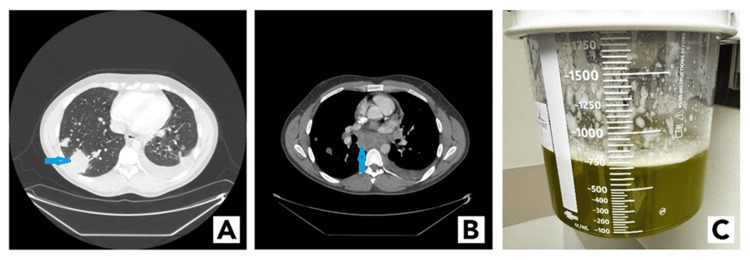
(A) Computed tomography of the chest, lung windows depicting a right lower lobe lung nodule (blue arrow) and a left-sided pleural effusion. (B) Computed tomography of the chest, mediastinal windows depicting a large subcarinal lymph node (blue arrow). (C) Green pleural effusion obtained from the patient.
